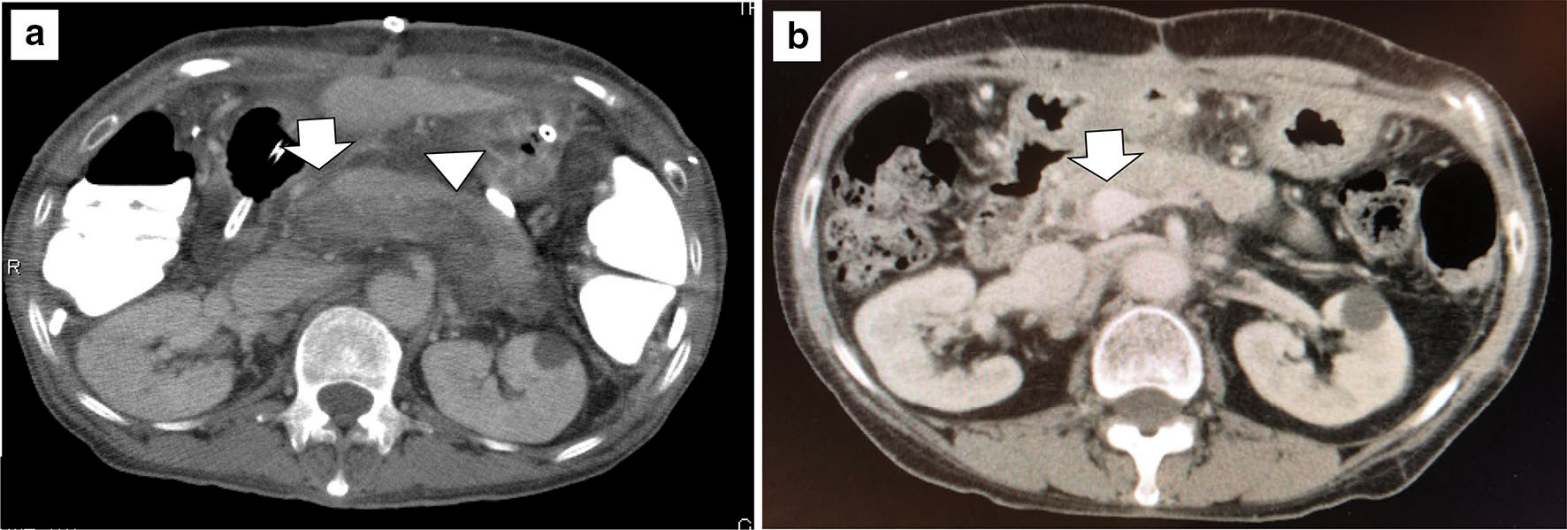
Representative case of portal vein thrombosis (PVT). a The PVT (arrow) extended from the splenic vein (arrowhead) stump 3 days after living donor liver transplantation (LDLT) with simultaneous splenectomy. b Enhanced computed tomography (CT) shows a patent portal vein (arrow) 1 year after LDLT
